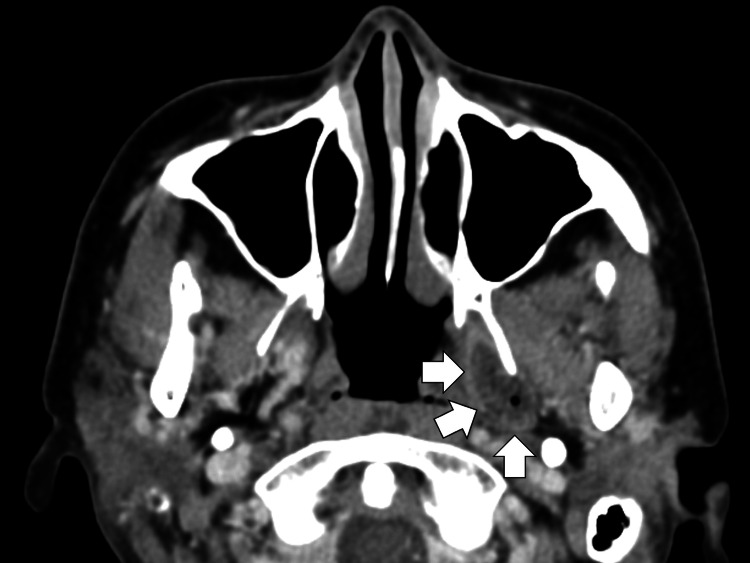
Contrast-enhanced head computed tomography showing a hypodense area with intralesional air and surrounding contrast-enhanced hyperdensity, indicative of a left parapharyngeal space abscess (white arrows).
